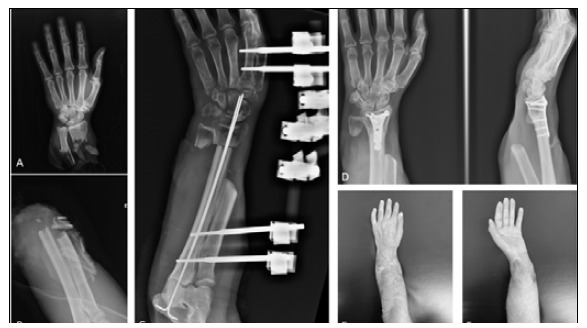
Case 6: (A, B) X-ray of the amputated limb on arrival at the hospital; (C) Radiography after three months of reimplantation showing no bone consolidation; (D) Image after eight years of surgery and a synthesis revision with good consolidation; (E) Clinical image of the limb after eight years.
